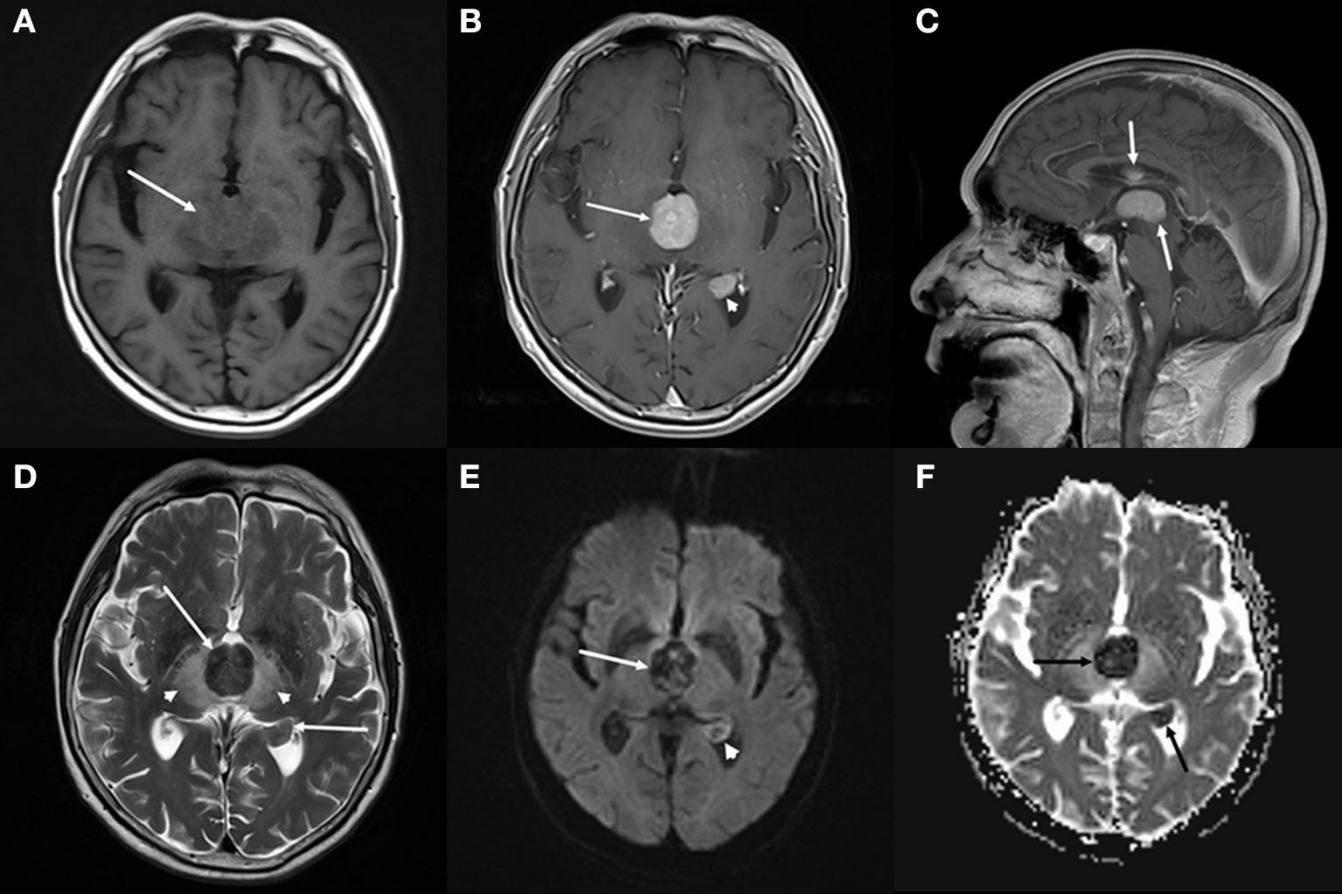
Brain magnetic resonance imaging (MRI). (A–C) Axial T1-weighted imaging (T1WI) (A) shows that the masses with slight hypointensity are in the third ventricle (arrow) and the posterior horn of the left lateral ventricle. Both of them are significantly enhanced on axial (B) and sagittal (C) T1WI with contrast. (D–F) T2-weighted imaging (T2WI) (D) shows the isointense to slightly hypointense masses (arrows), the swollen and hyperintense parenchyma around the third ventricle (arrowheads). Diffusion-weighted imaging (DWI) (E) shows a hypointense mass in the third ventricle (arrow) and a slightly hyperintense mass in the lateral ventricle (arrowhead), both of them with low apparent diffusion coefficient (ADC) value (ADC value: 0.425 × 10^3^*mm*^2^/s, 0.628 × 10^3^*mm*^2^/s) (F, arrow), all suggesting diffusion restricted.
